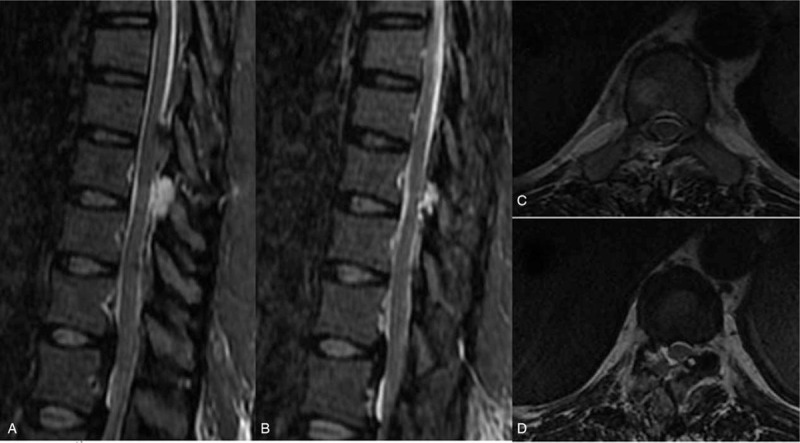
Magnetic resonance imaging on the first day after surgery: decompression of the T9 to 10 spinal canal was sufficient.
